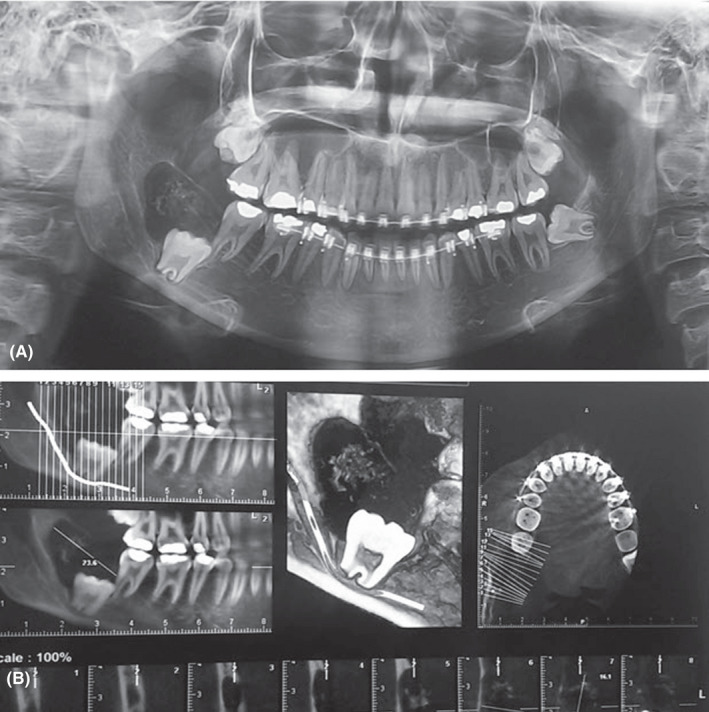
Panoramic radiograph of the lesion that shows well‐defined radiolucent lesion contained areas of radiopacity around the crown of an unerupted third molar (A). Cone beam computed tomography (CBCT) view of the lesion (B)
